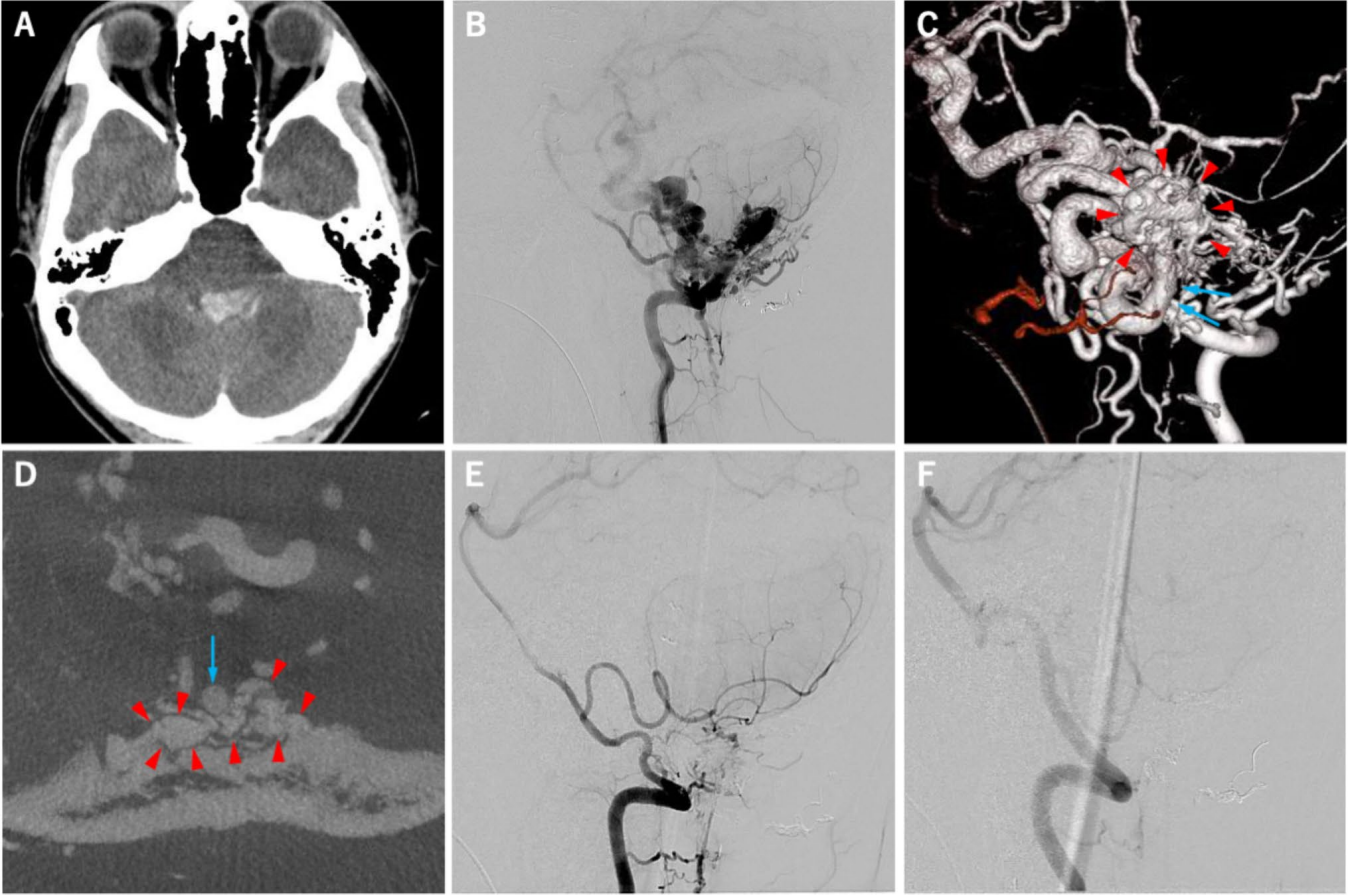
A 17-year-old male presented sudden headache followed by consciousness disturbance. The CT image on admission showed the SAH and dense IVH in the fourth ventricle (a). The lateral view of right vertebral VAG revealed high-flow dAVF at FMR with the remarkable dilatation of the posterior fossa veins (b). Posterior-anterior view of 3-dementional rotational right VAG demonstrated shunted pouch on the dura matter (red arrowhead) and the BV (blue arrow) that working as a single drainer of dAVF (c). The BV (blue arrow) and shunted pouch on the inner surface of dura matter (red arrowhead) was demonstrated by the axial image of cone-beam CT (d). This patient underwent 2-session of TAEs followed by surgical interruption via midline-suboccipital craniectomy with the same fashion as in case 1. The lateral images of right (e) and left (f) VAG obtained after surgery showed the complete disappearance of high-flow dAVF
